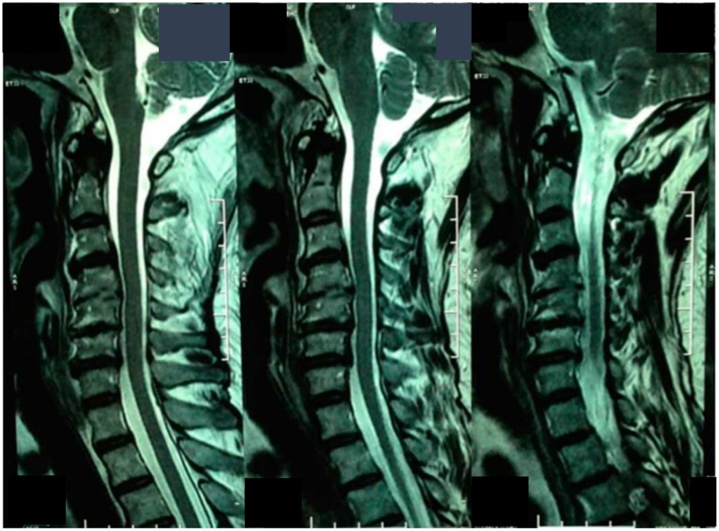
Sagittal magnetic resonance imaging showing narrowing of the spinal canal.
